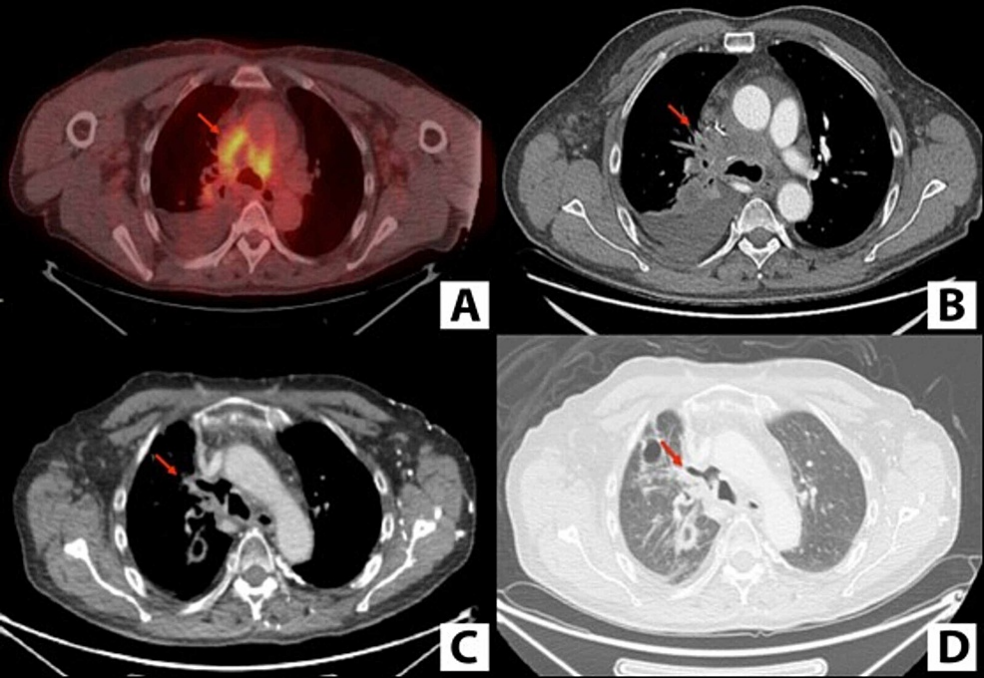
Positron emission tomography showing fluorodeoxyglucose-avid right paratracheal mass (A) and computed tomography showing right paratracheal mass (B). Computed tomography mediastinal window (C) and lung window (D) showing air in the mediastinum.
